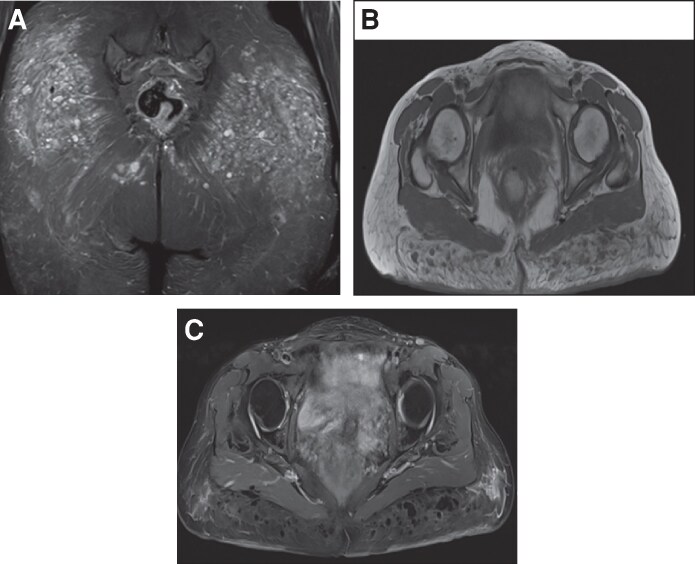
Pelvic MRI of a 58-year-old female patient. (A) Coronal slice, Short TI inversion Recovery (STIR) sequence. (B) axial slice, T_1_-weighted sequence. (C) axial slice, proton density sequence. Multiple nodular images are observed, hyperintense on the STIR sequence (A) and hypointense on the T_1_-weighted and proton density sequences (B and C), with a maximum diameter of 1 cm. These lesions are randomly distributed throughout the subcutaneous tissue, fascia, and some superficial muscle fibers of the right gluteus maximus muscle. Based on the clinical history, they correspond to nonresorbable exogenous material.
